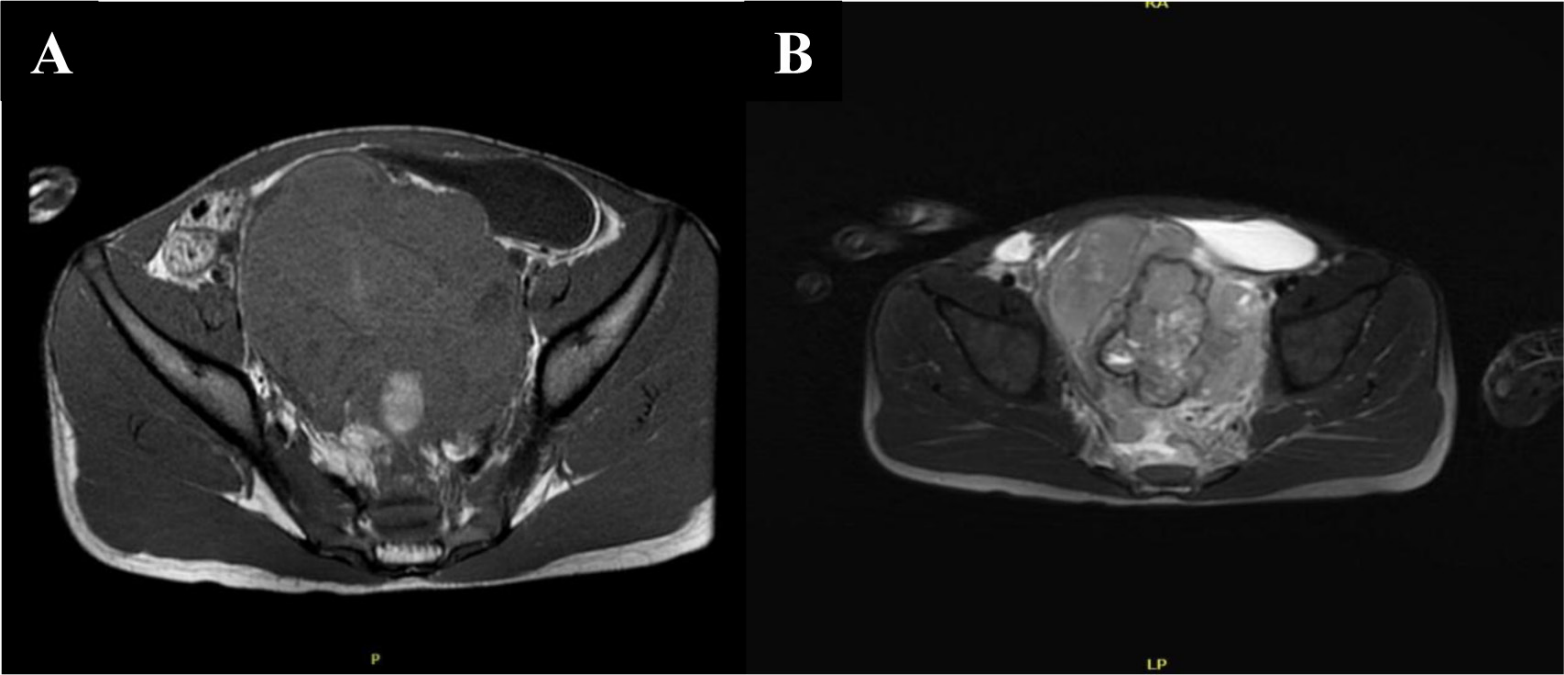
Axial T1-weighted MRI image (A) shows a well-defined, heterogeneous low signal intensity and lobulated pelvic mass with focal hyperintensies indicating hemorrhagic zones. Axial T2-weighted image (B) shows a hyperintense and heterogeneous content.
